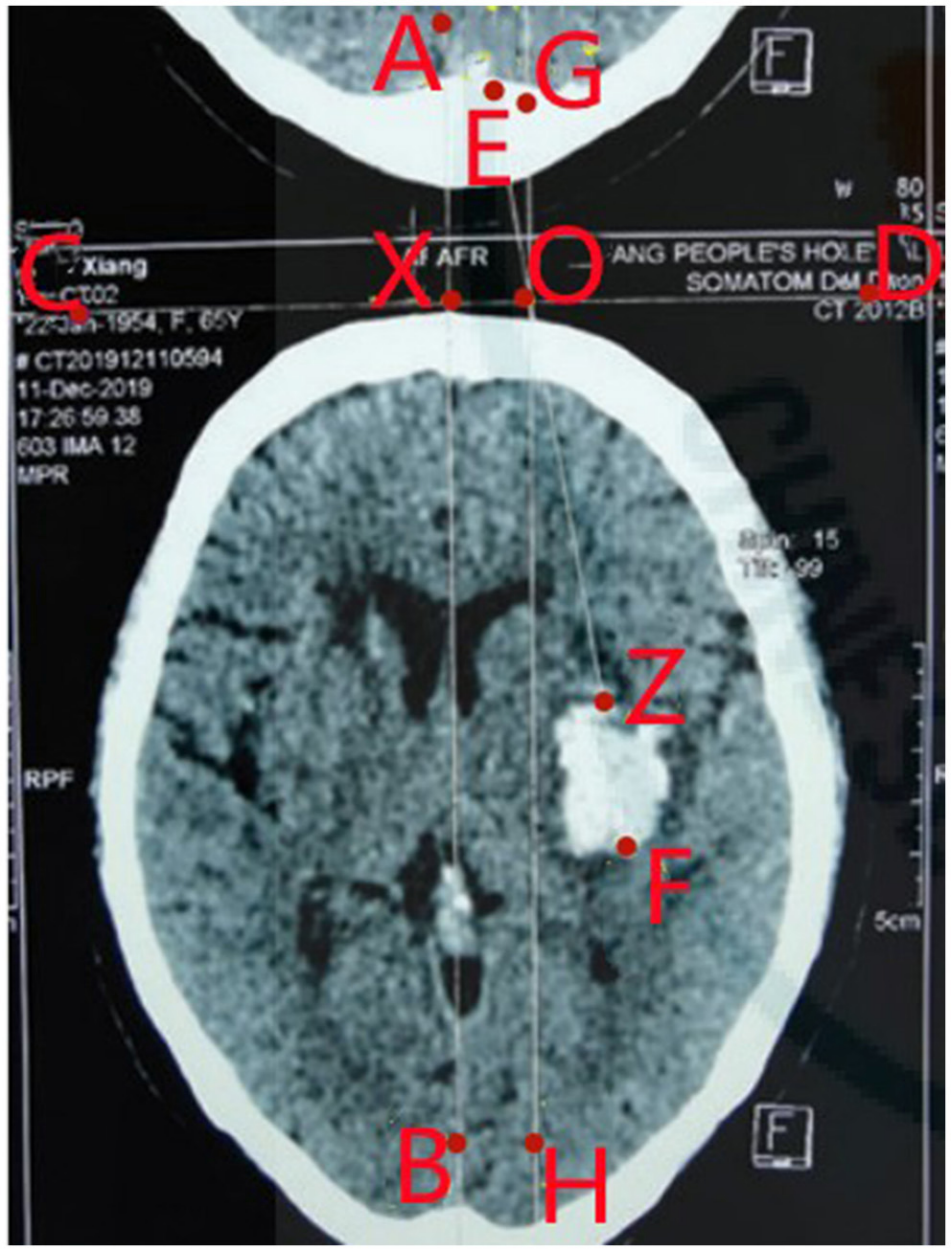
The cerebral CT image. A: A point on the midline (vertical line) of the CT image; B: Another point on the midline of the CT image; C: A point on the vertical line (horizontal line) of the midline AB, the horizontal line is close to the scalp; D: The midline AB A point on the vertical line (horizontal line) of the puncture track; E: a point on the reverse extension of the puncture tract OF; F: The most distal end of the hematoma; G: A point on the vertical line GH, GH is perpendicular to the horizontal line CD, the vertical point is Point O; X: The intersection of the vertical line AB and the horizontal line CD; O: The puncture point, which is a point on the horizontal line CD, and the line segment XO is 1.5 cm; Z: The intersection of the puncture tract OF and the hematoma.
